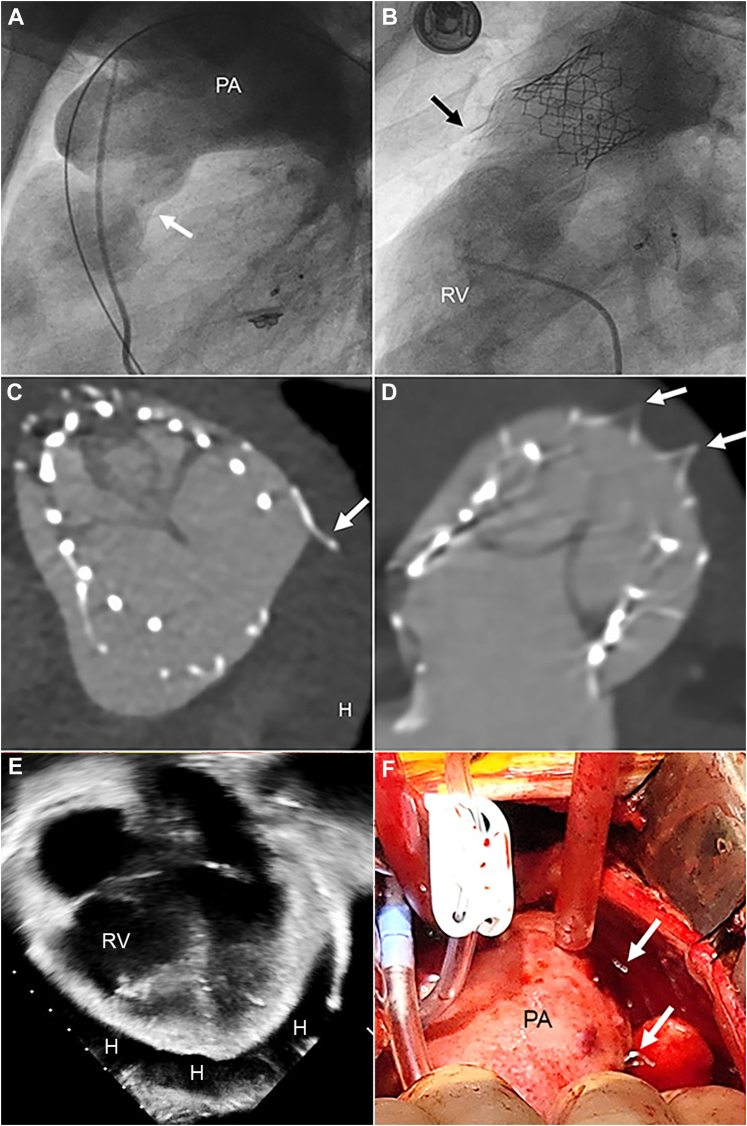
Patient 1 (A) This pulmonary arterial (PA) angiogram in the lateral projection demonstrates severe pulmonary regurgitation and the intended landing zone for the Alterra Adaptive Prestent in patient 1 (the arrow indicates the annulus). (B) This post-implant angiogram in the right ventricle (RV) demonstrates the Alterra Prestent and SAPIEN 3 valve in the supra-annular position. The proximal tines of the Alterra Prestent appear to be extravascular (arrow). (C and D) These (C) axial and (D) coronal cardiac computed tomography images obtained the day after implant demonstrate perforation of the distal tines of the Alterra Adaptive Prestent through the anteroleftward wall of the main PA (arrows), along with a moderate hemopericardium (H). (E) This 4-chamber echocardiographic image the day after implant demonstrates a large hemopericardium (H). (F) This intraoperative photograph reveals that the distal tines of the Alterra Prestent have perforated the left side of the main PA (arrows).
